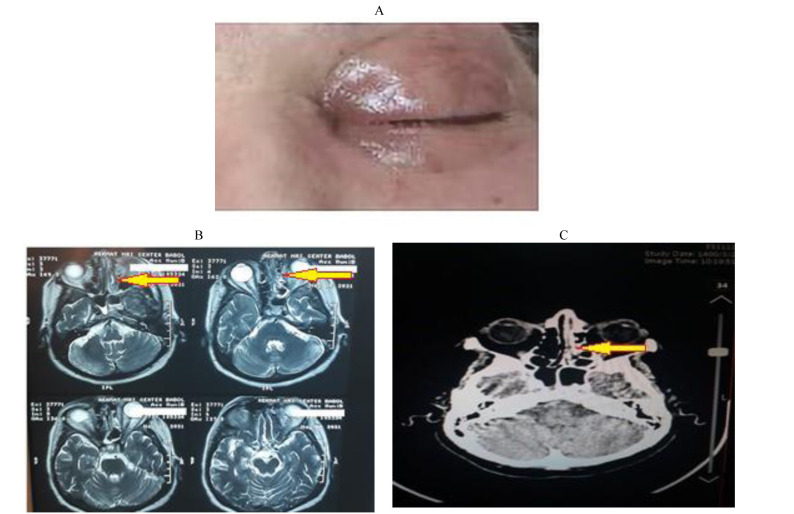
A; Preoperative presentation of left eye proptosis and chemosis; B; MRI scan image showing the involvement of left eye; C; MRI scan image showing the involvement of paranasal sinuses
